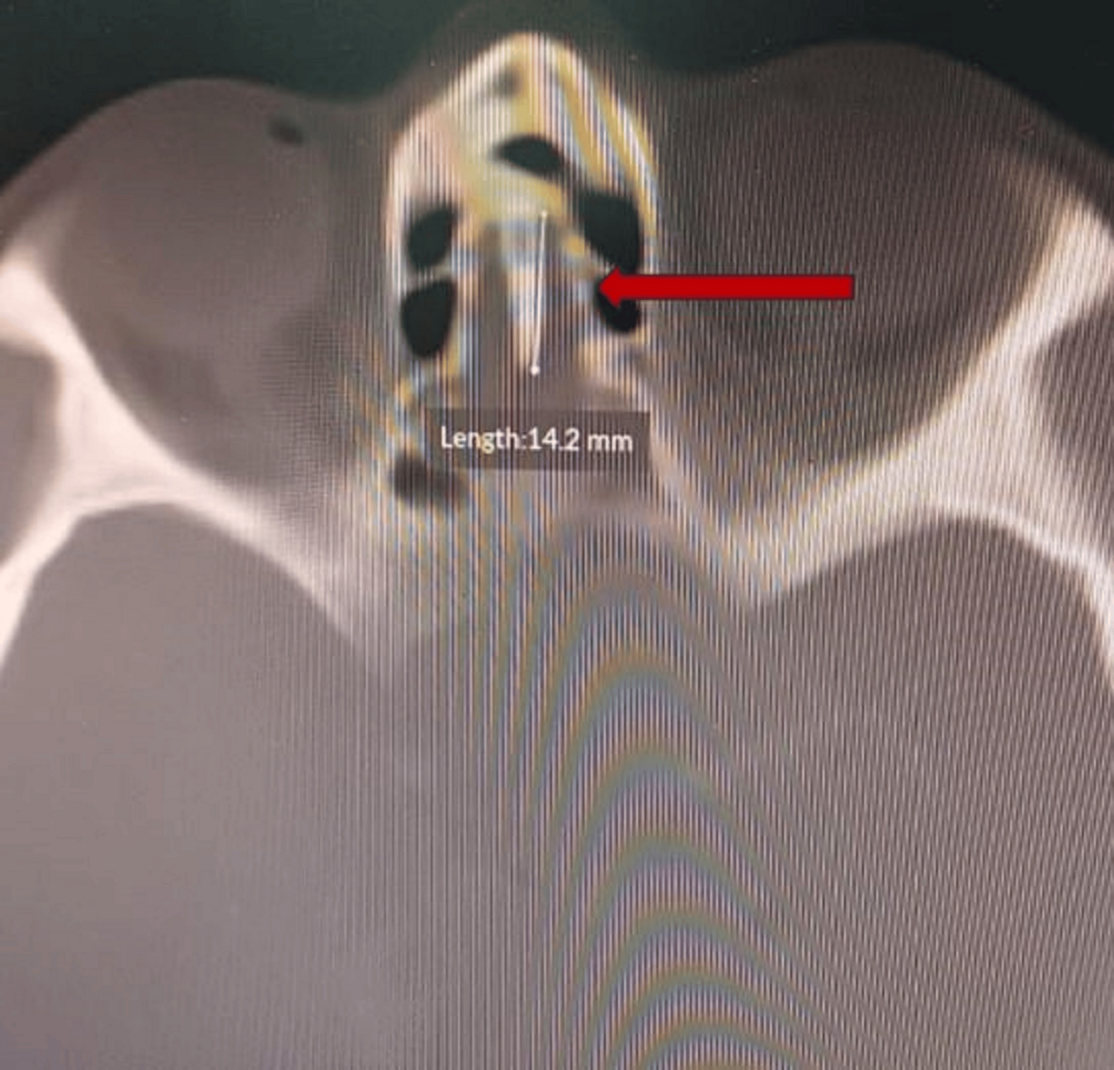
Axial section of CT scan paranasal sinuses showing left sided type 2 Yenigun
